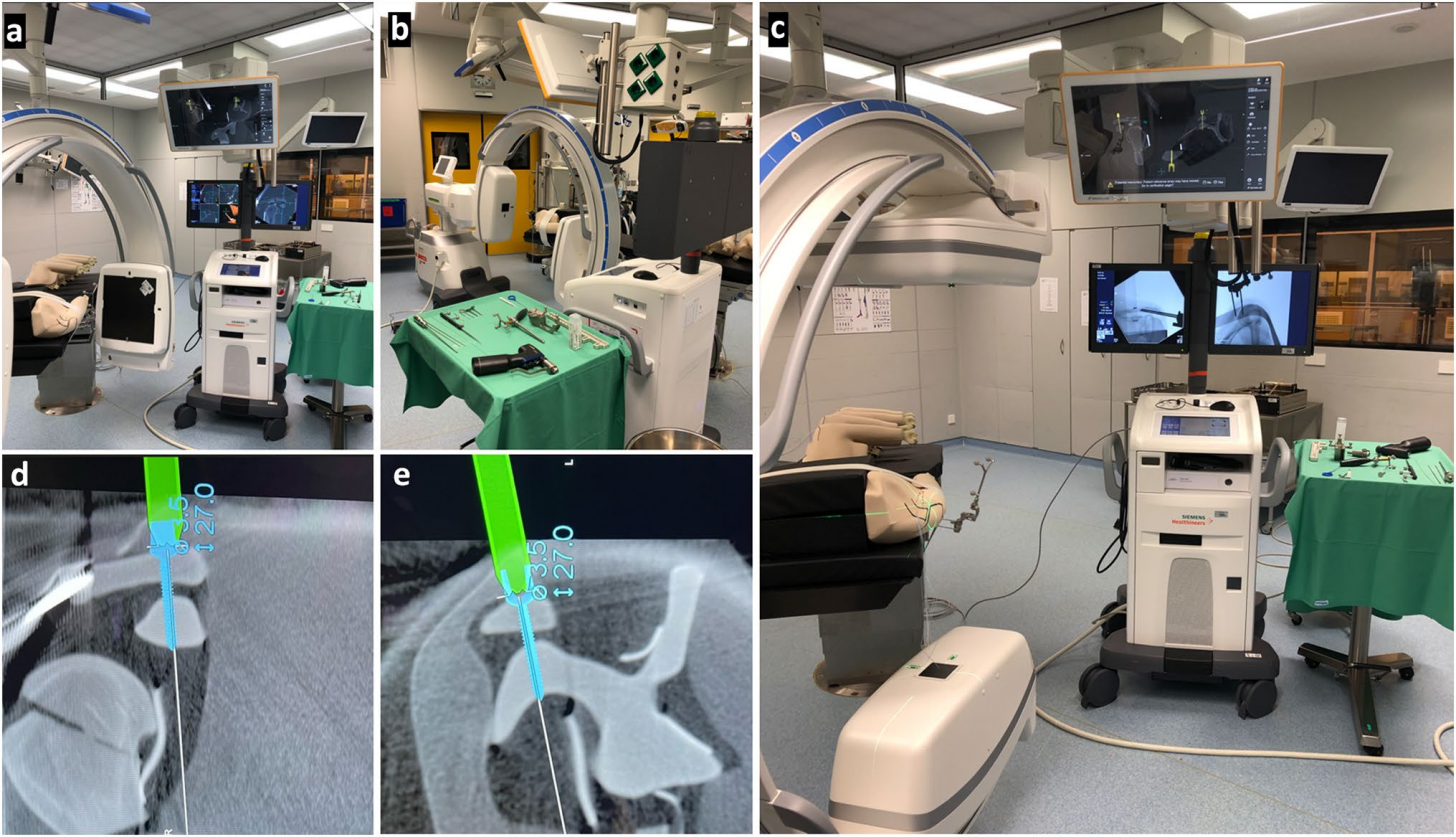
System setup in the operating room. a–c a synthetic shoulder model is attached to the operating table. The mobile C-arm is placed in parasagittal position at the head end. The navigation camera is pointed at the references on the model and the C-arm. The navigation monitor still shows images of the first DVT scan with a planned CC drill channel. On the X-ray monitor, the already implanted SD is visible in two planes. The drill, wires, and pointers are on the instrument table. d–e the planning of the navigated drill channel in CC direction is shown enlarged in two planes (coronal and sagittal)
